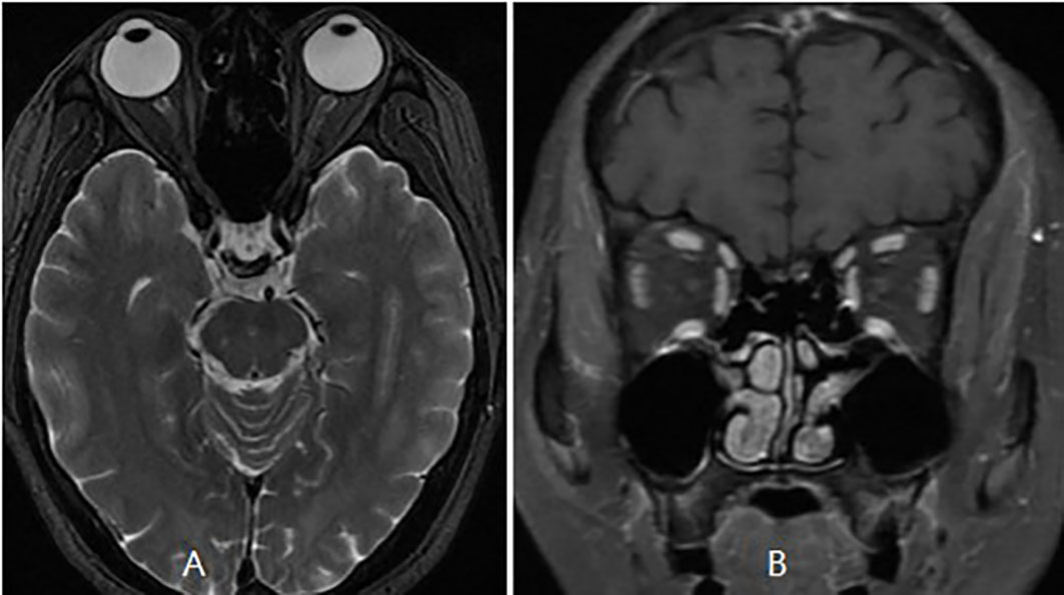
Orbital MRI showed normal optic nerves (A) with no enhancement (B).
